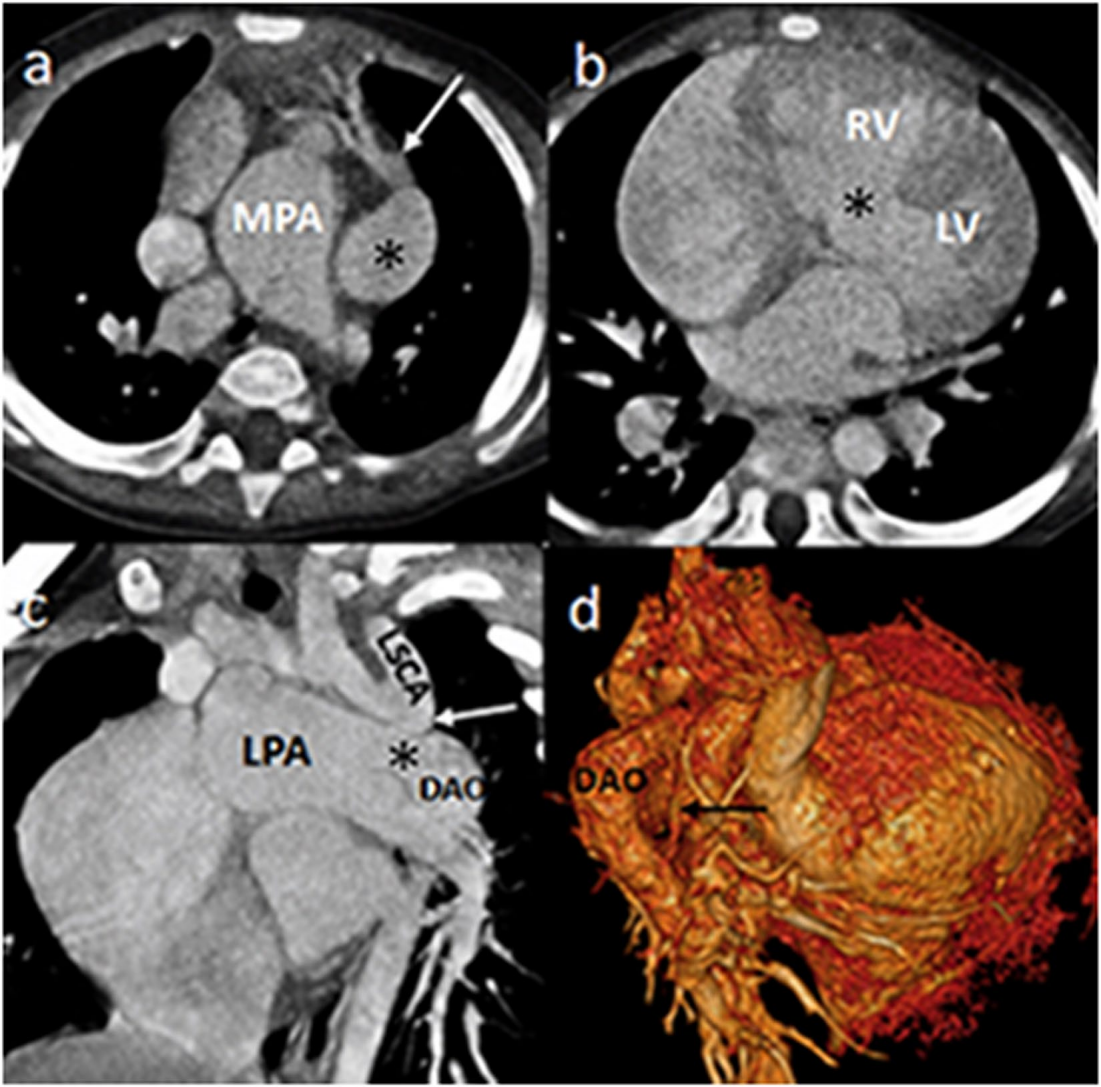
DSCT images of an 1-year-old boy with interrupted aortic aorta. The axial image (a) presents that the dilated right coronary aorta (white arrow) abnormally origins from the dilated left coronary sinus (asterisk) and distributes tortuously. The axial image (b) shows a large ventricular septal defect (asterisk). The multiplanar reformatted image (c) displays the aortic arch terminates at LSCA (white arrow). A ductus arteriosus (asterisk) extends from the LPA to the DAO. Volume rendering image (d) shows the collateral vessel (black arrow). *Abbreviations*: DSCT, dual-source computed tomography; MPA, major pulmonary artery; LV, left ventricle; RV, right ventricle; LSCA, left subclavian artery; LPA, left pulmonary artery; DAO, descending aorta.
